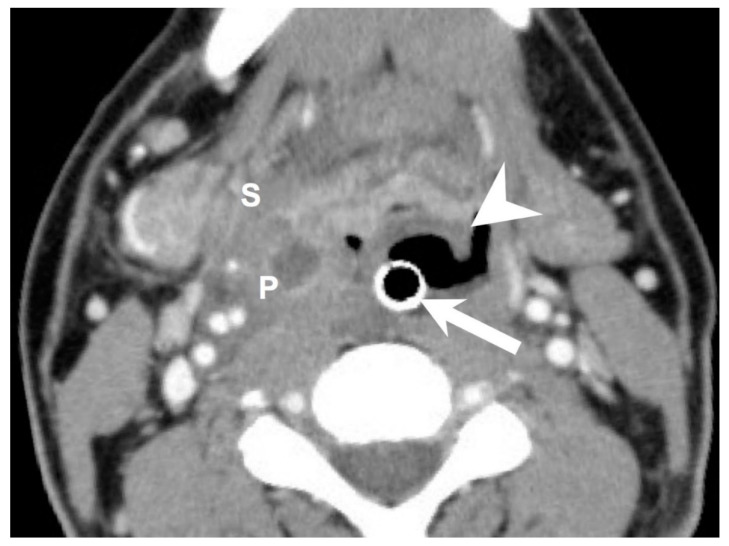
Axial CT view of an intubated patient with concurrent acute epiglottitis and multiple deep neck infections. Abscesses were detected in the parapharyngeal space and submandibular space. Arrow: endotracheal tube insertion; arrowhead: swollen and inflammatory epiglottis; P: parapharyngeal space; S: submandibular space. 300 × 300 DPI.
